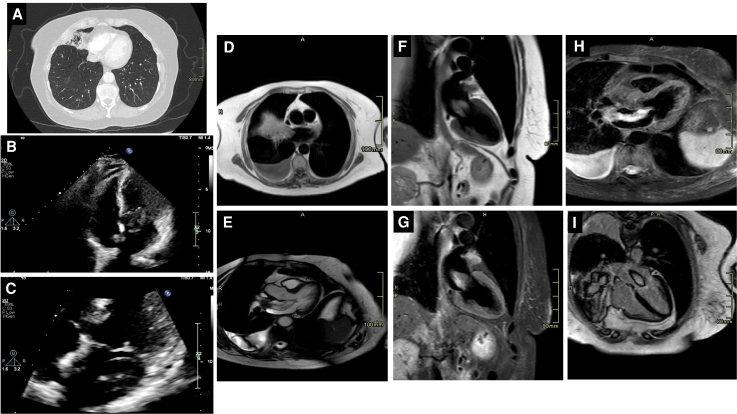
(*A*) The initially performed CT thorax showing what initially appeared to be an intra-atrial thrombus. (*B*) Transthoracic echocardiogram four-chamber left apical view showing a large left atrial highly mobile mass (30 × 17 mm) appearing to be attached by a stalk to the interatrial septum partially protruding into the left ventricle through the mitral valve. (*C*) Focused view of the left atrial mass described in *B*. (*D*) Cardiac MRI imaging (horizontal long axis) demonstrating a large right middle lobe lung mass with direct continuity into the right upper pulmonary vein and extension into the left atrium. The absence of discrete atrial wall attachment argues against myxoma. (*E*) Cardiac MRI horizontal plane with early and late gadolinium enhancement, showing no contrast uptake within the left atrial component of the mass. Lack of enhancement excludes organized thrombus (which typically demonstrates peripheral enhancement) and supports malignant extension. (*F*) Cardiac MRI vertical long axis view showing the intracardiac mass prolapsing into the mitral valve in diastole. The smooth prolapse without obstruction and absence of atrial wall tethering further distinguish tumour extension from myxoma. (*G*) Cardiac MRI T1- and T2-weighted sequences of the left atrial mass demonstrating intermediate T1 and high T2 signal intensity, with no suppression on fat-saturated imaging—features consistent with soft-tissue tumour rather than thrombus. (*H*) Cardiac MRI horizontal plane imaging showing mass continuity from the lung into the left atrium via the pulmonary vein. Despite diastolic prolapse across the mitral valve, there was no functional valve compromise. (*I*) Cardiac MRI late gadolinium enhancement sequence demonstrating absence of uptake within the mass, helping to exclude thrombus (expected peripheral enhancement) and supporting tumour invasion as the most likely diagnosis.
